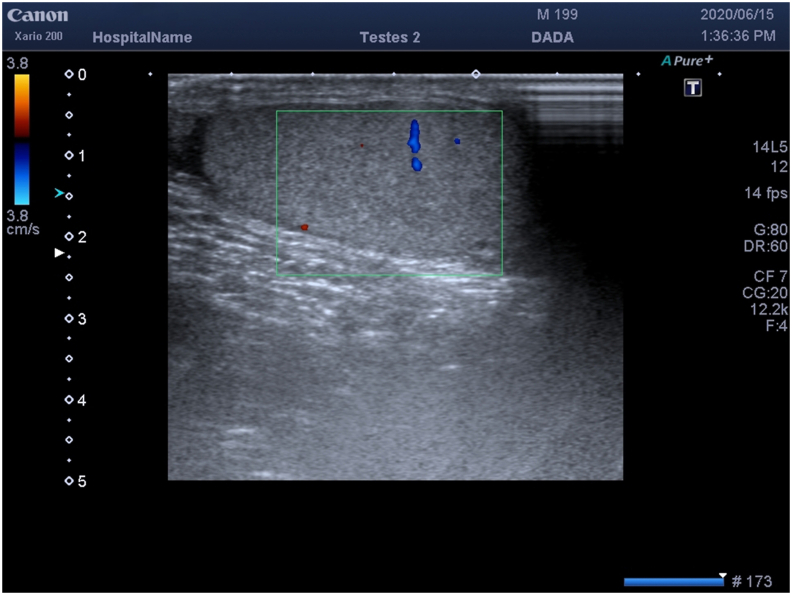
Color Doppler image shows blood flow and echotexture of the supernumerary testis, which is similar to the other testes. (For interpretation of the references to colour in this figure legend, the reader is referred to the Web version of this article.)
